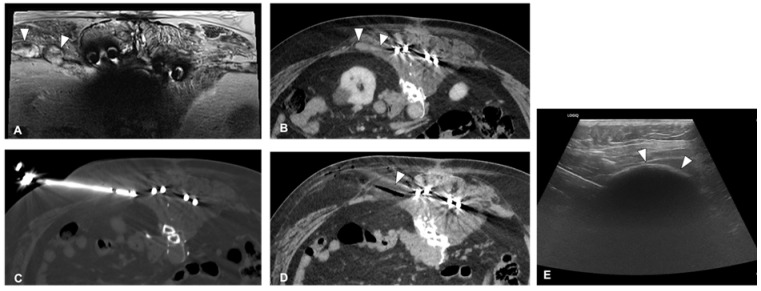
A 55-year-old man, cryoablation in a recurrent chordoma showed in an axial T2 MR image ((A)—arrowheads) and axial postcontrast CT image ((B)—arrowheads), for local tumor control. The cryoprobe was placed into the lesion under CT (C) and US guidance, with subcutaneous hydro dissection performed to protect the skin. The ice ball encompassed the entire lesion (D). Also, note the aspect of the ice ball on ultrasound ((E)—arrowheads).
